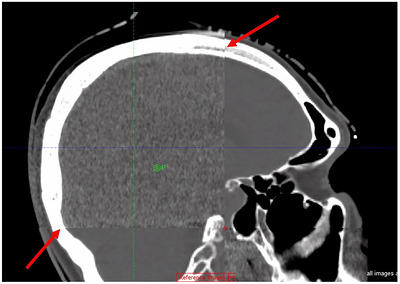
Sagittal view of the CT and CBCT scans of a cranial patient. The two scans have been registered using rigid registration and displayed using the same window level (bone). The interval between the acquisitions of the two scans is less than a week. Nonetheless, mismatch in the skull is observed, highlighted by the red arrows. Given the rigidity of the skull, this mismatch likely originates from image distortion rather than anatomical variation.
